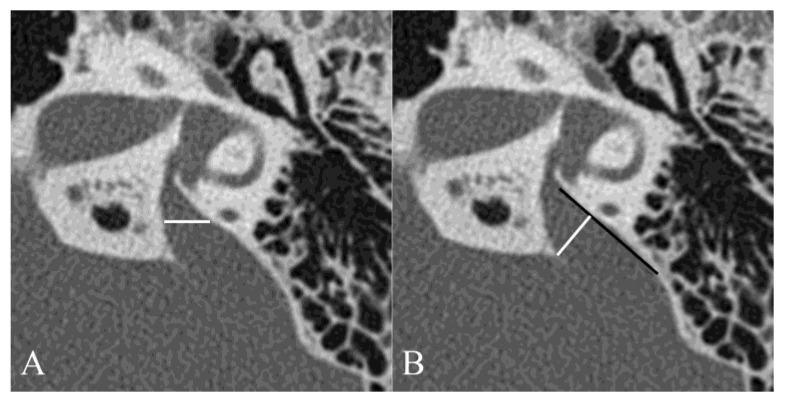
Measuring the VA midpoint and porous width in axial computed tomography images. The VA midpoint width (white line) was measured at the half the distance from VA fundus to its external pore (A). The VA porous width (white line) was measured from the opercular margins to the spots on the posterior temporal bone walls (black line) whose surface was perpendicular to the measurement lines (B).
